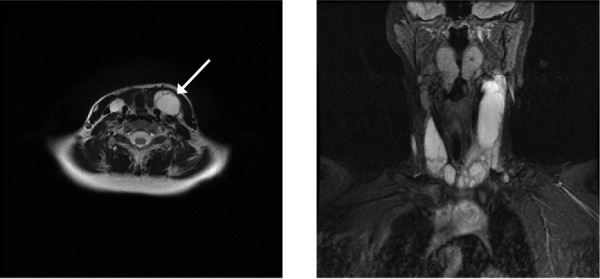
Contrast-enhanced T2-weighted MRI of patient’s neck. Axial (left) and coronal (right) MR imaging of the patient’s neck reveals a peripherally enhancing, multi-septated, cystic lesion arising in the superior mediastinum and extending bilaterally in the neck. The lesion lies deep to the sternocleidomastoid muscles and anterior to the carotid sheaths.
